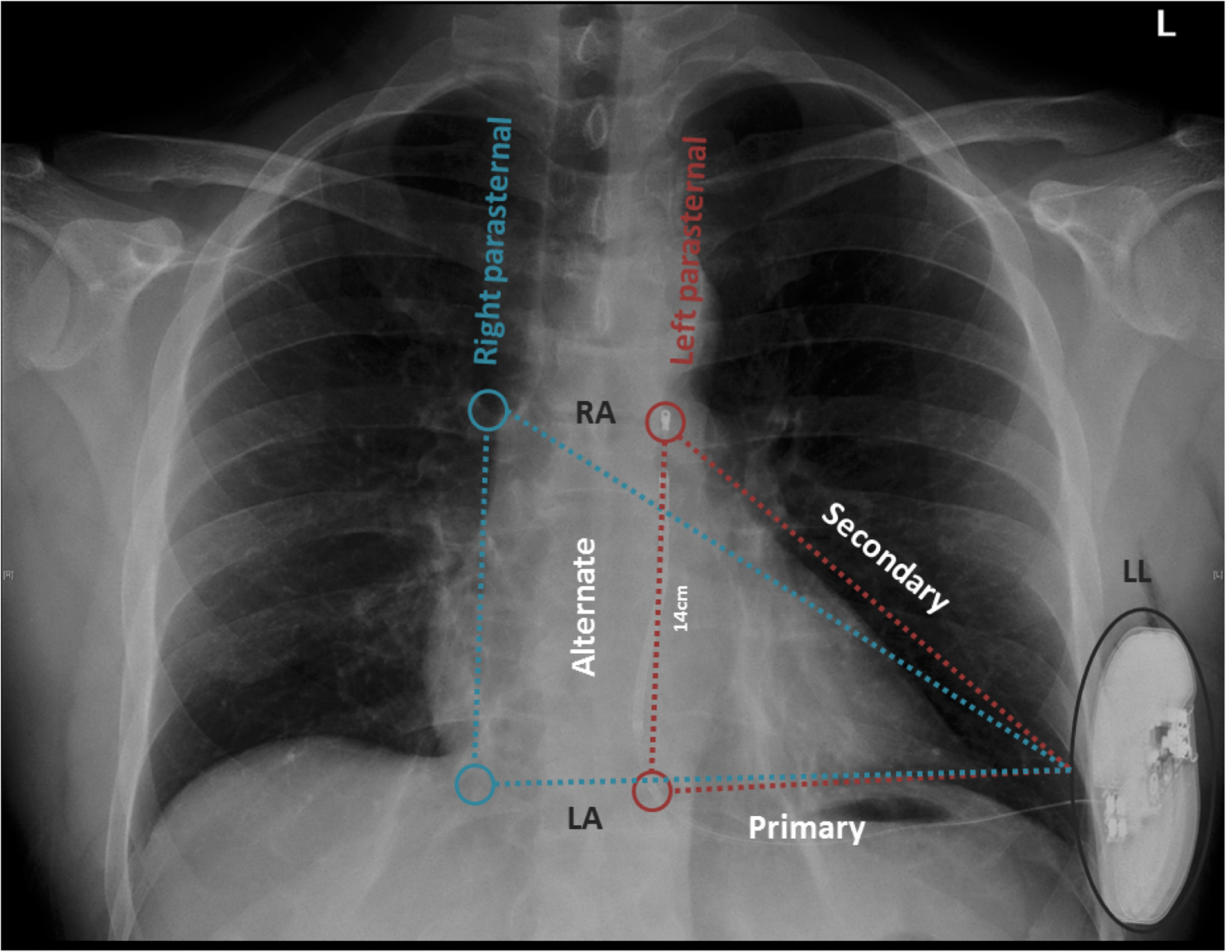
Chest X-ray of a patient with an implanted S-ICD in the left parasternal position. The 3 possible vectors formed from the S-ICD lead and the S-ICD can are depicted. Red colored are the vectors formed in the left parasternal position and blue colored are the vectors formed in the right parasternal position. RA, right arm; LA, left arm; LL, left leg
